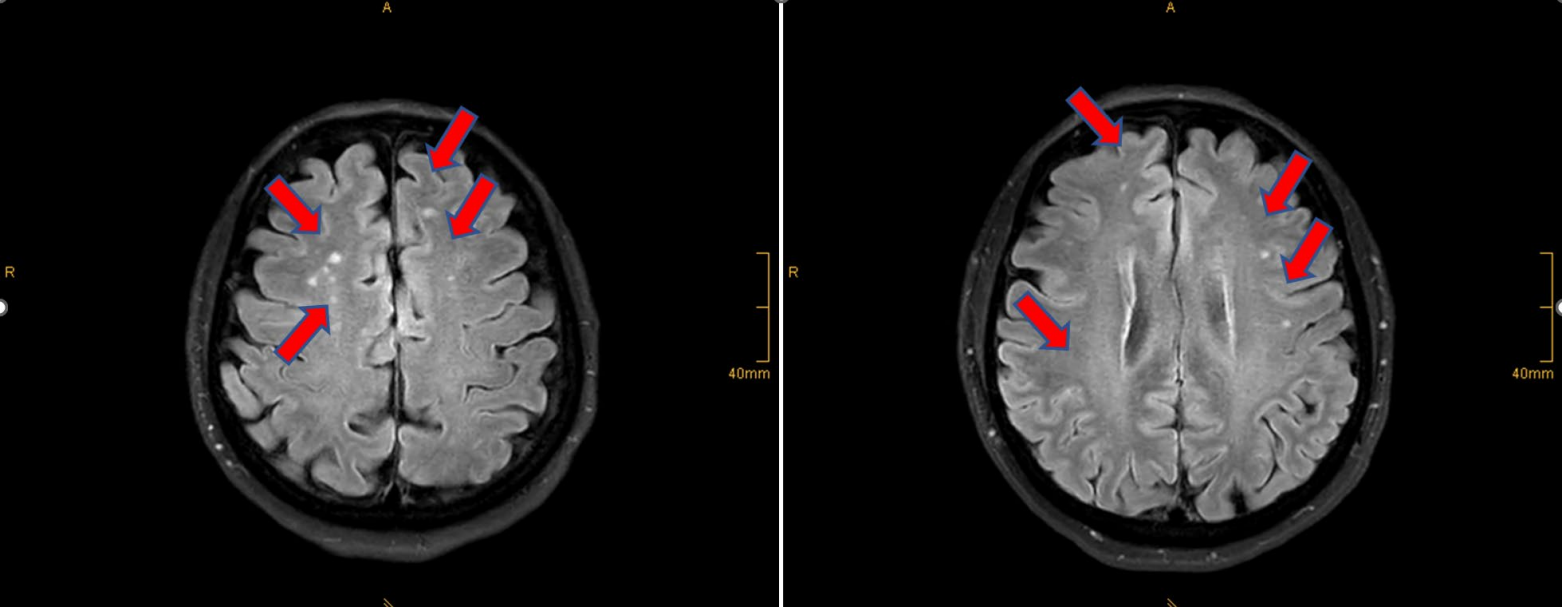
Brain MRI of an individual with the Flammer syndrome phenotype; the marked multi-site lacunar microinfarction is asymptomatic; the image is adapted from [11]
